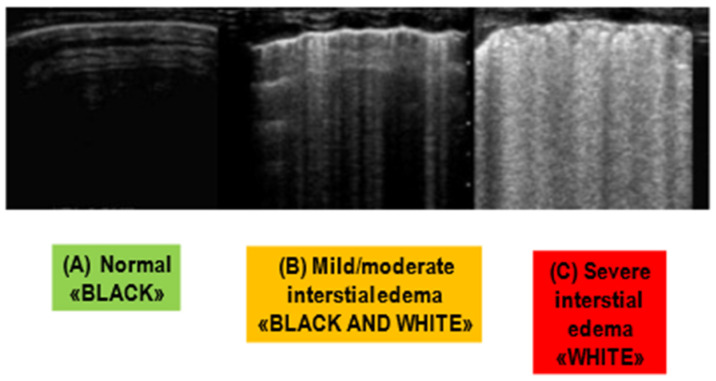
Lung Ultrasounds (LUS). In the normal state (A), there are no B-lines. In a state of mild to moderate interstitial edema (B), an overall hypoechoic appearance is maintained, with sparse B-lines forming. As the edema progresses to become more clinically severe (C), the hypoechoic appearance is lost, with a high density of B-lines.
